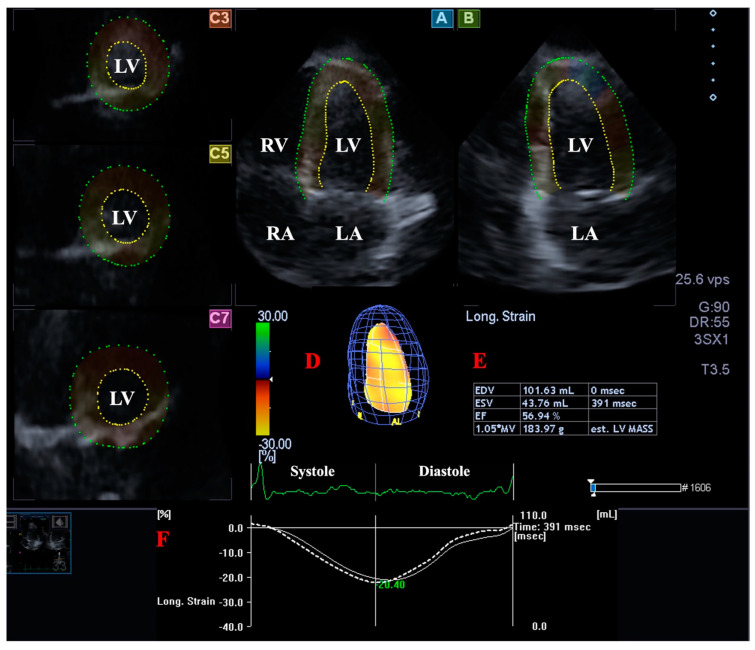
Three-dimensional (3D) speckle-tracking echocardiography-derived left ventricular (LV) volumetric analysis. Apical four-chamber view (A); apical two-chamber view (B); short-axis view at basal (C3), midventricular (C5), and apical LV level (C7) are demonstrated together with a 3D cast of the LV (D) and calculated LV volumetric data (E). Time-global LV longitudinal strain curve (white line) and time-LV volume change curve (dashed white line) are shown as well (F). Abbreviations. LA: left atrium, LV: left ventricle, RA: right atrium, RV: right ventricle, EDV: end-diastolic volume, ESV: end-systolic volume, EF: ejection fraction.
